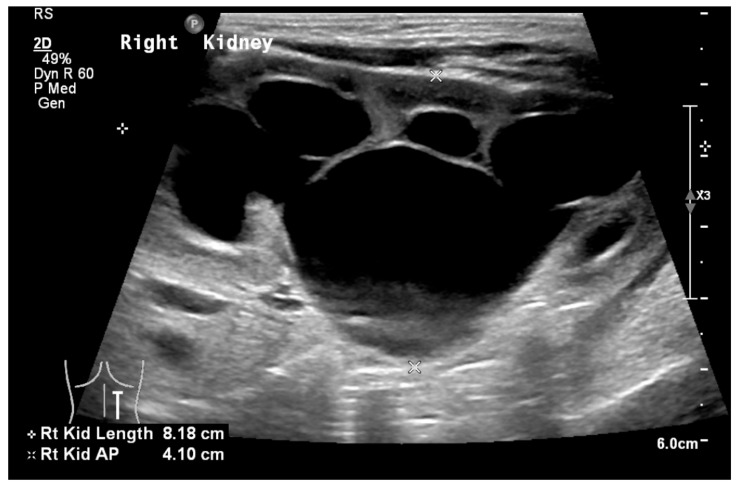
Renal ultrasound (US) showing hydronephrosis in a 5-day-old male infant with ureteropelvic junction obstruction.
